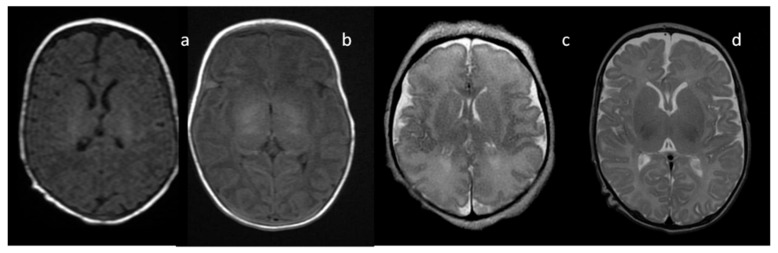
Infant brain MRI—no differences in signal intensity between the basal ganglia and the surrounding structures before the completion of myelination: (a,b) axial T1-weighted images (T1-WI) in a neonate with a hyperintense signal in the posterior internal capsule; (c) axial T2-weighted images (T2-WI) at the 36th week of gestation; (d) axial T2-WI in a neonate.
